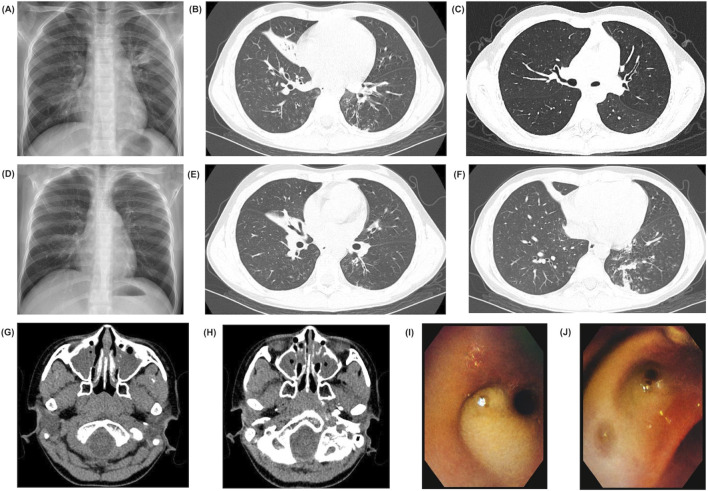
Radiological evolution and bronchoscopic findings of the proband. (A–C) Imaging obtained 5 months prior to the current admission. (A) Chest X-ray showing patchy opacities in the right lung. (B,C) Chest CT showing consolidation in the right middle lobe, with bronchiectasis in the left lung, indicating chronic airway damage. (D–F) Imaging upon current admission. (D) Chest X-ray with persistent high-density shadows in the right lower lung field. (E,F) Chest CT showing segmental consolidation, predominantly in the right middle lobe and left lingular segment. (G,H) Paranasal sinus CT: diffuse mucosal thickening and opacification of bilateral maxillary and ethmoid sinuses, confirming the diagnosis of pansinusitis. (I,J) Fiberoptic bronchoscopy: endoscopic view of the right middle and lower lobe bronchi showing mucosal hyperemia, edema, and obstruction of the lumen by copious purulent secretions.
